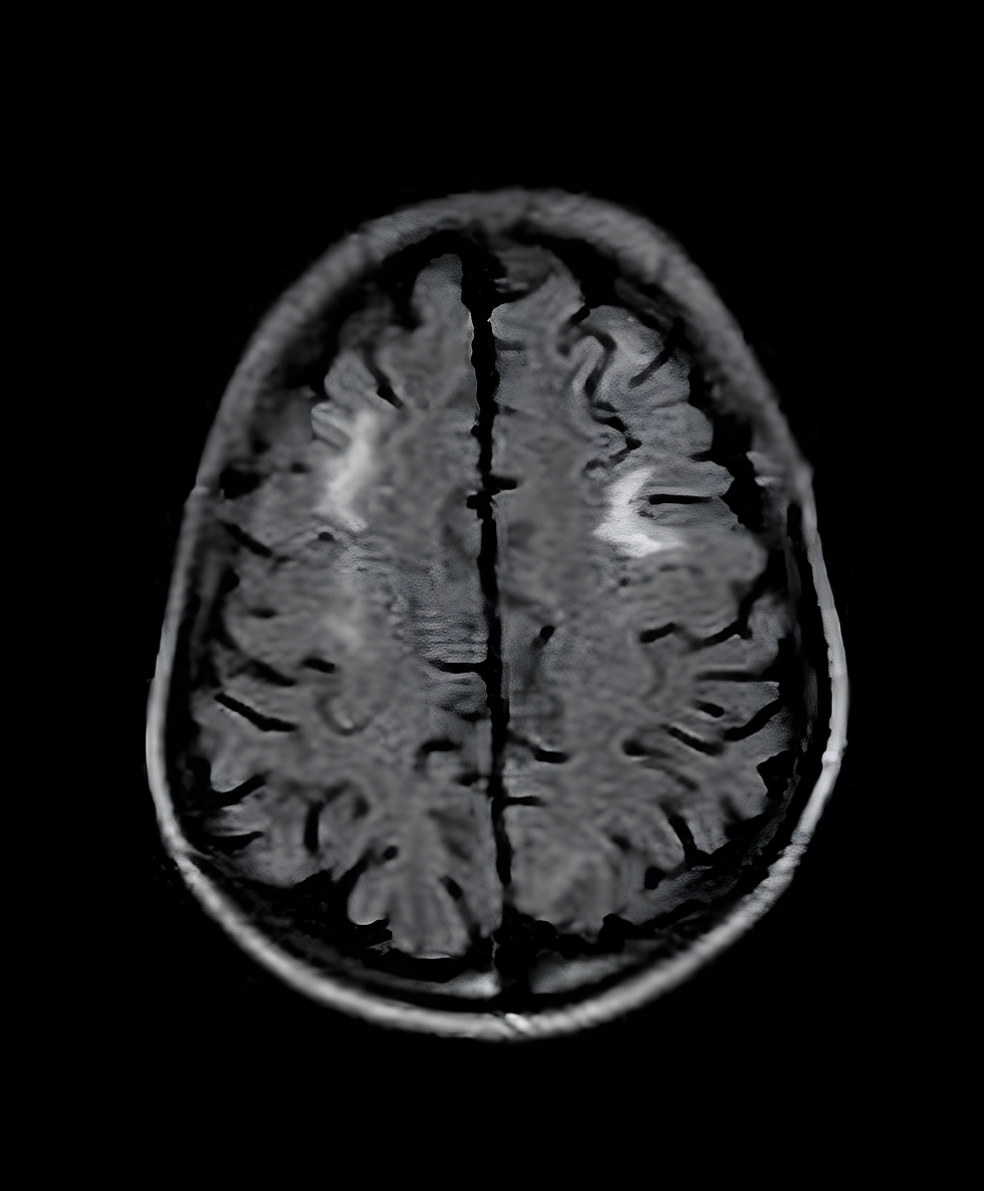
Axial, non-enhanced T1-weighted MRI image demonstrating bilateral multiple juxtacortical white matter high signal intensity lesions MRI: magnetic resonance imaging
